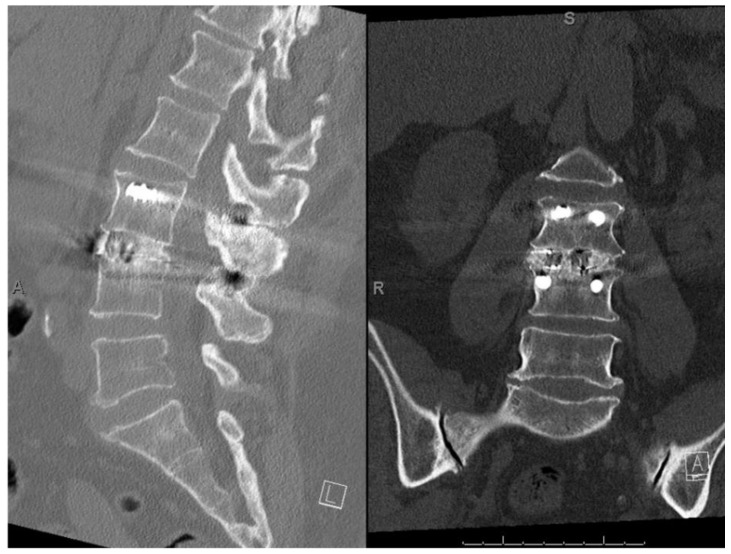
Spinal fusion success. One-year postoperative CT scan demonstrating successful bridging bone on sagittal and coronal reconstructions.
